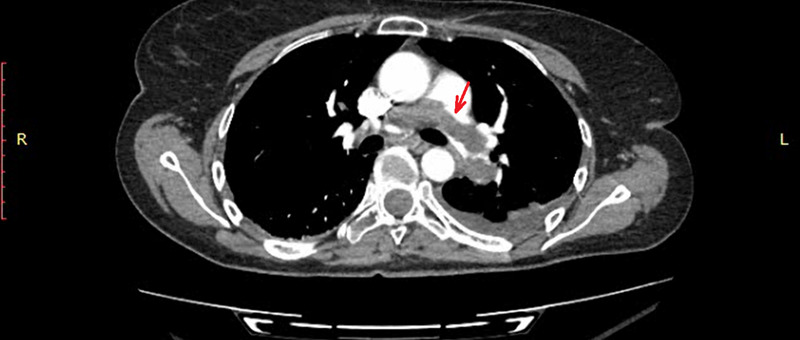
Thoracic CT scan with contrast It showed a massive pulmonary thromboembolism in both pulmonary arteries, having a saddle-like appearance (arrows).
